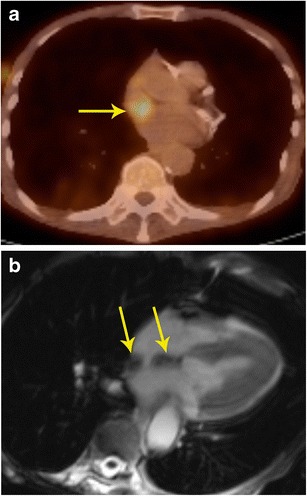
Localization of abnormal activity. a Axial FDG-PET/CT image shows a focus of high uptake adjacent to the SVC, which extended inferiorly. b Four-chamber SSFP MR image through the heart shows lipomatous hypertrophy of the interatrial septum (arrows), with sparing of fossa ovalis. This corresponds to the area of hypermetabolism in PET/CT. Although a benign lesion, this can occasionally show uptake in FDG-PET
